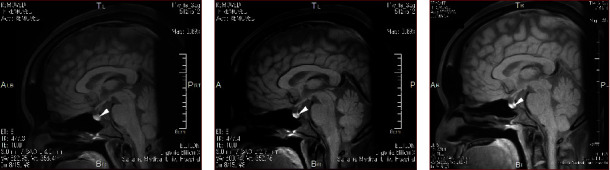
Fat-suppressed T1-weighted magnetic resonance imaging of the hypothalamic-pituitary area. (a) A magnetic resonance imaging (MRI) scan on the fourth day after the delivery showed no obvious abnormal findings in the hypothalamic-pituitary area, except for a loss of the normal bright spot in the posterior lobe (arrowhead). (b) An MRI on the 28th day after the delivery showed a partial reappearance of this bright spot (arrowhead). (c) An MRI on the 201st day after the delivery showed a clear bright spot in the posterior lobe (arrowhead).
